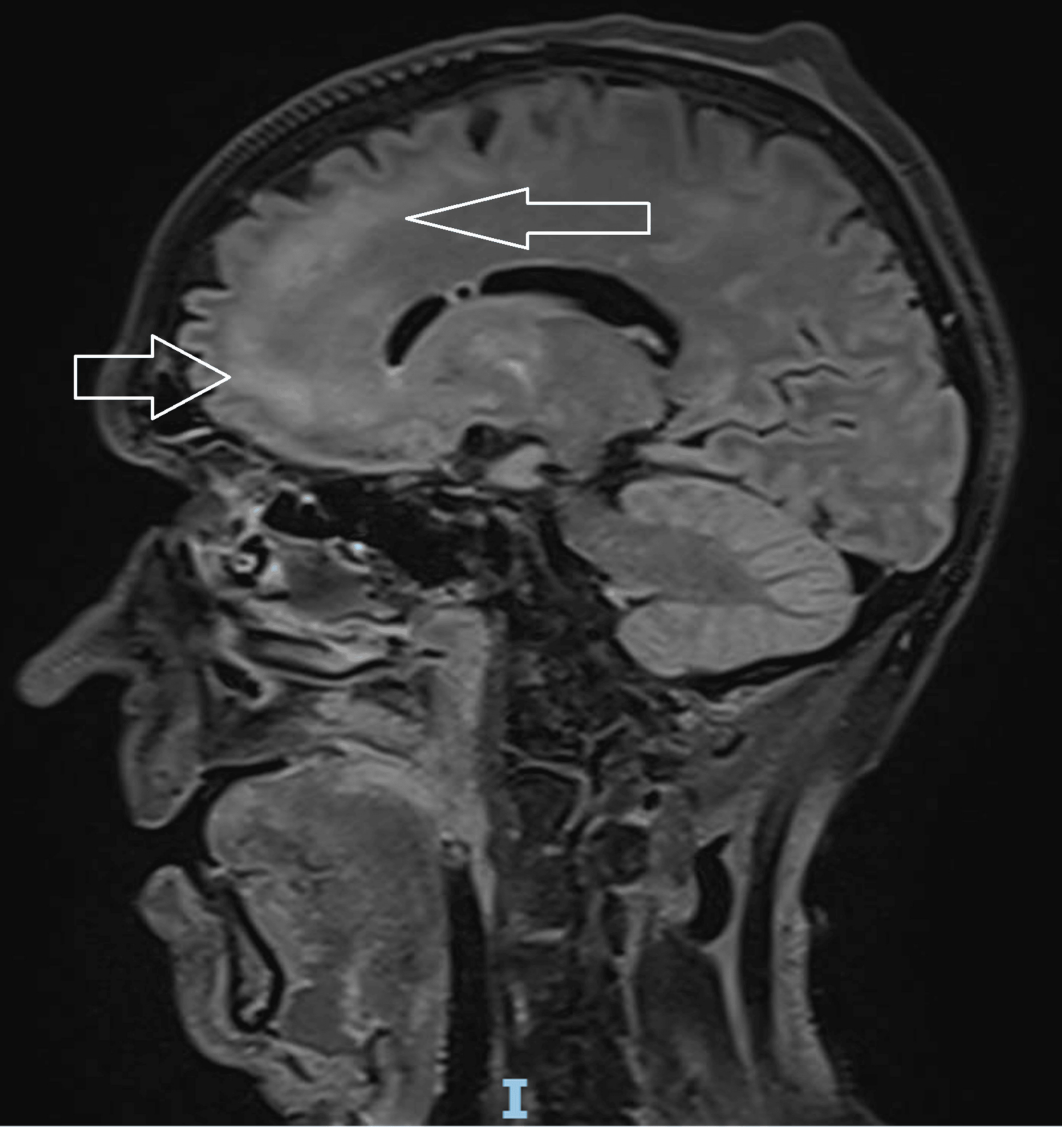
MRI head T2 sagittal view showing T2 hyperintensity throughout the supratentorial white matter.
